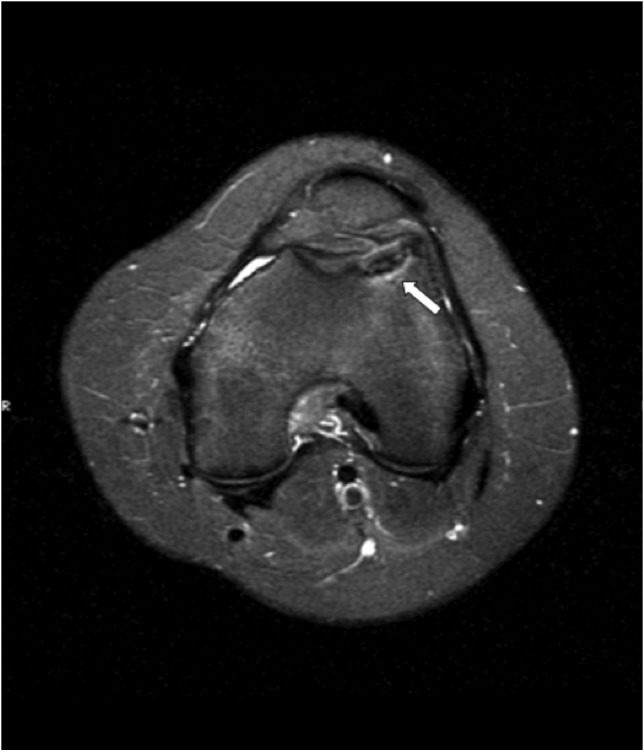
Preoperative magnetic resonance image demonstrates the fragment in situ with fluid signal and bone marrow edema under the lesion” (white arrow).
